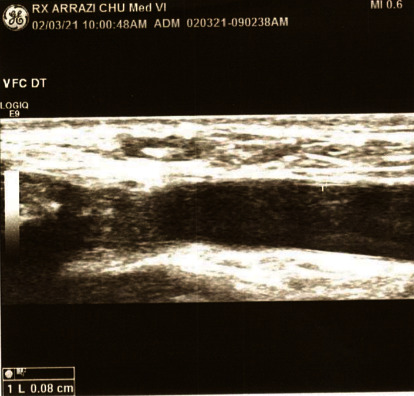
Image showing sonographic measurement of the thickness of the right common femoral vein wall at 0.8 mm.
